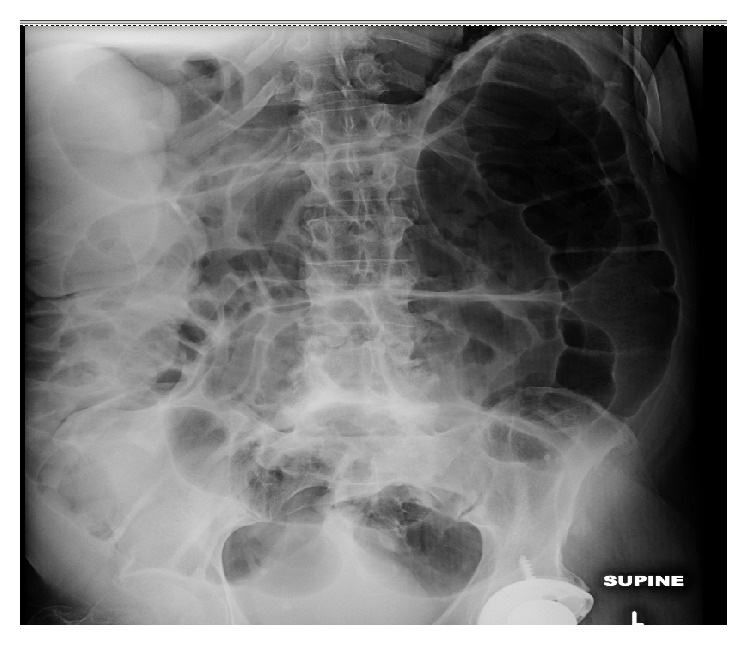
Abdominal X-ray showing severe colonic dilation due to pseudoobstruction.
